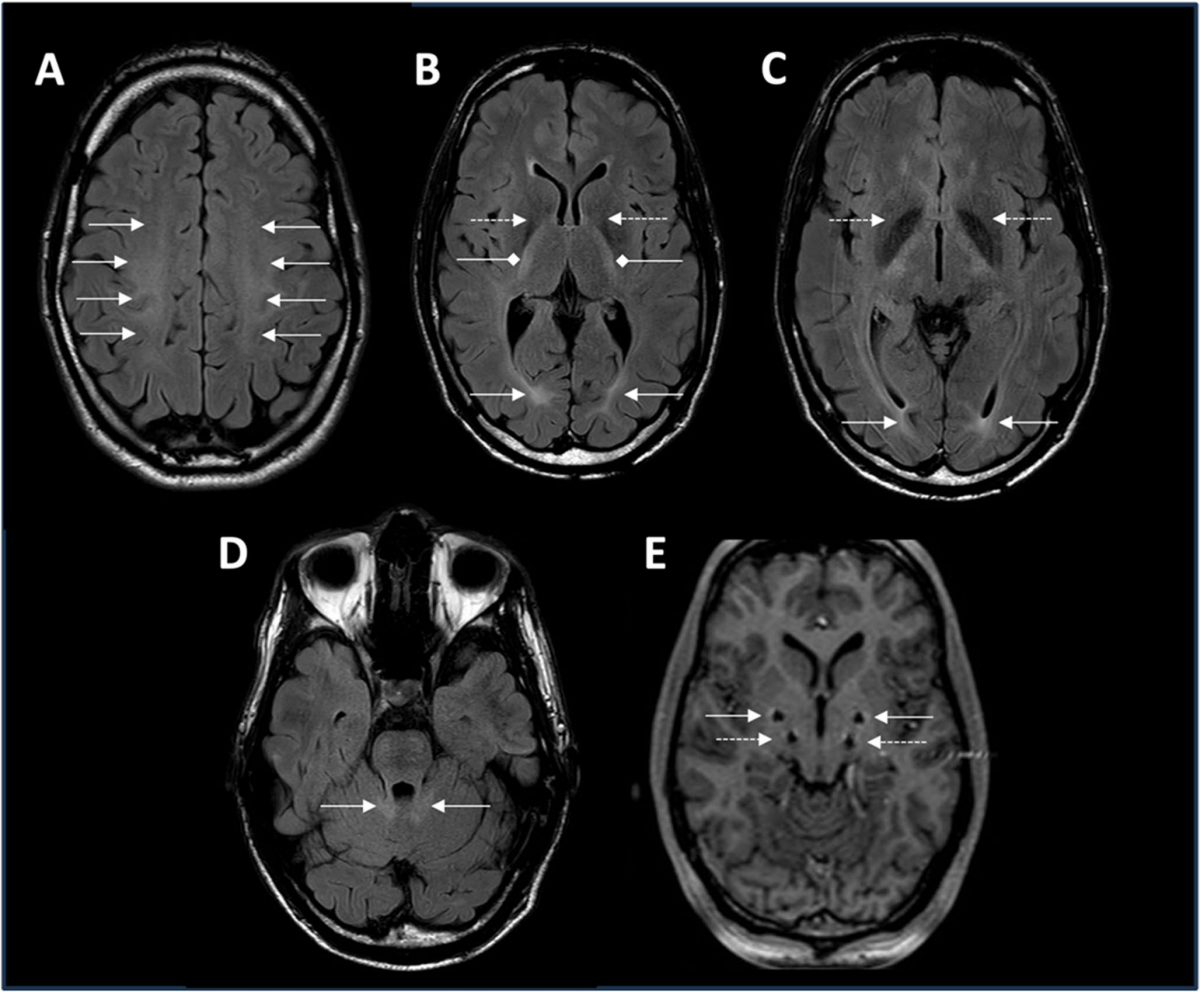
MR imaging. A-D: MRI from the reported patient at the age of 27 years. Axial T2-weighted (fluid-attenuated inversion recovery) images are shown. Imaging demonstrated symmetrical T2-hyperintense cerebral demyelination involving central (white arrows in A) and occipital (white arrows B and C) white matter, the internal capsule (white square-ended arrows in B), and the cerebellar peduncles (white arrows in D). Moreover, symmetric T2 hypointensities in the globus pallidus were observed (white dotted-line arrows in B and C). E. Post-surgery MR imaging of the four deep brain stimulation electrodes bilaterally in the globus pallidus internus (GPi, white arrows) and nucleus ventralis intermedius (ViM, white dotted-line arrows)
